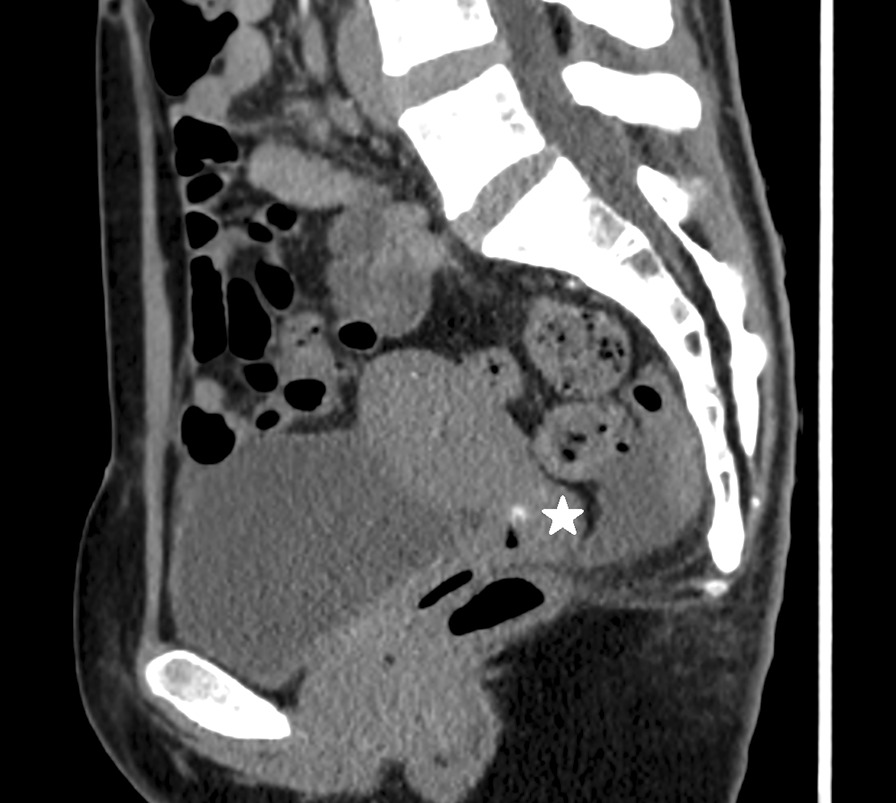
CT Angiogram prior to embolisation: Sagittal reconstruction of the pelvis on CT angiogram shows a small pseudoaneurysm (white star) of the uterine artery located at the cervical–vaginal junction
